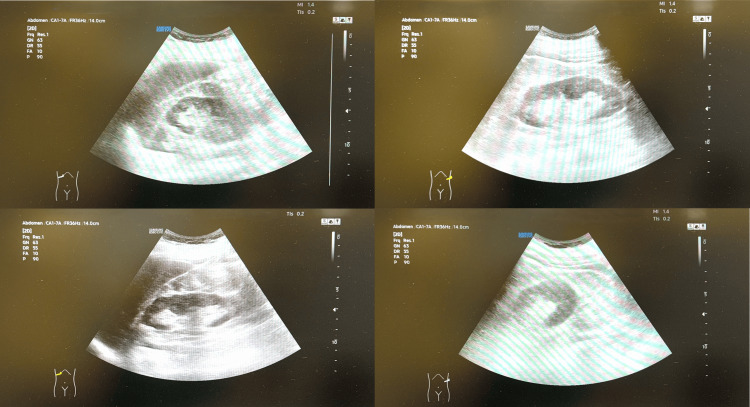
Ultrasound of the urinary tract showing normal kidneys.
